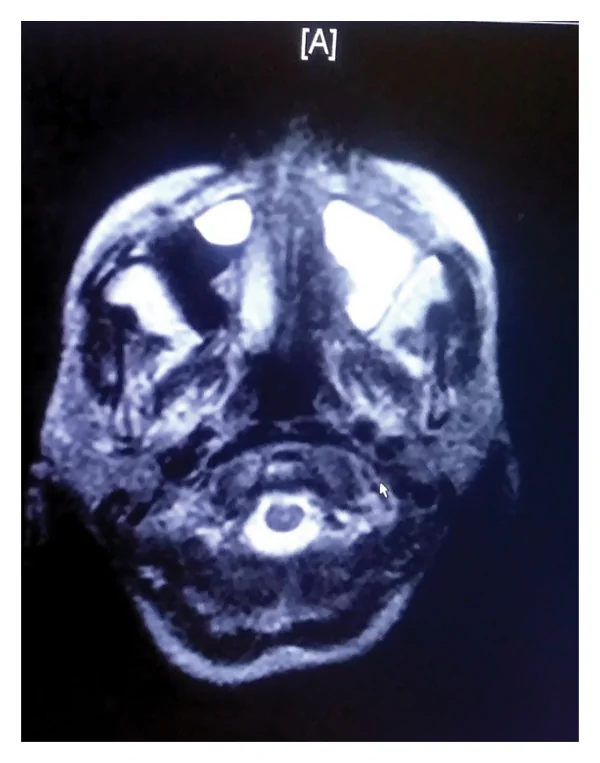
Coronal T2‐weighted magnetic resonance imaging (MRI) of the paranasal sinuses (anterior orientation indicated by [A] at top) demonstrating homogeneous hyperintense fluid within the left maxillary sinus, consistent with inflammatory sinus disease. No definite radiological features of invasive fungal sinusitis, orbital extension, or intracranial involvement were identified at the time of imaging.
